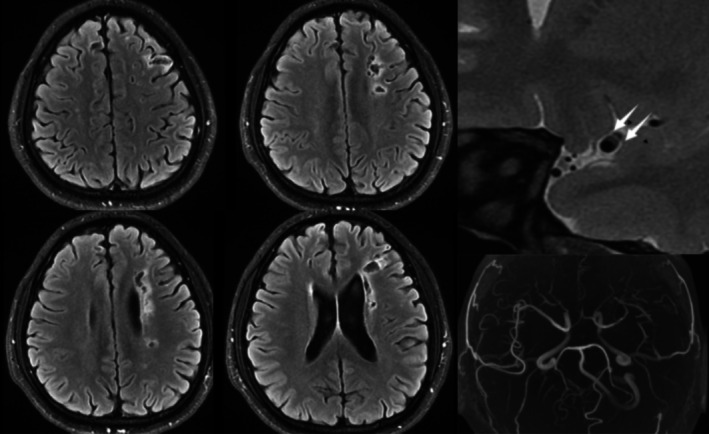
Twenty‐eight‐year‐old male patient with AS‐MMV presented with imaging findings including: Left watershed zone infarction on FLAIR sequence. HRMRI showed eccentric wall thickening, positive remodeling, and plaque signal in the left middle cerebral artery (MCA) (white arrow). TOF‐MRA revealed multiple focal stenoses in the left MCA along with signal decrease in bilateral anterior cerebral arteries, while both posterior cerebral arteries were well‐visualized.
